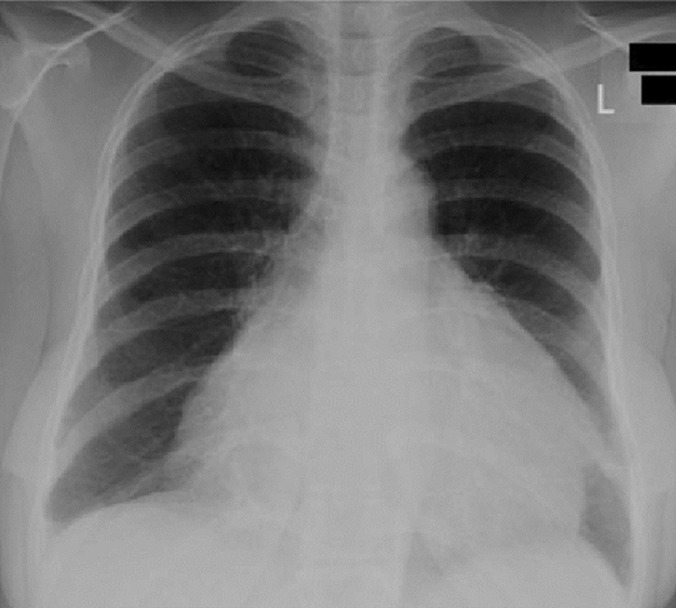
chest radiography demonstrating an enlarged heart shadow
